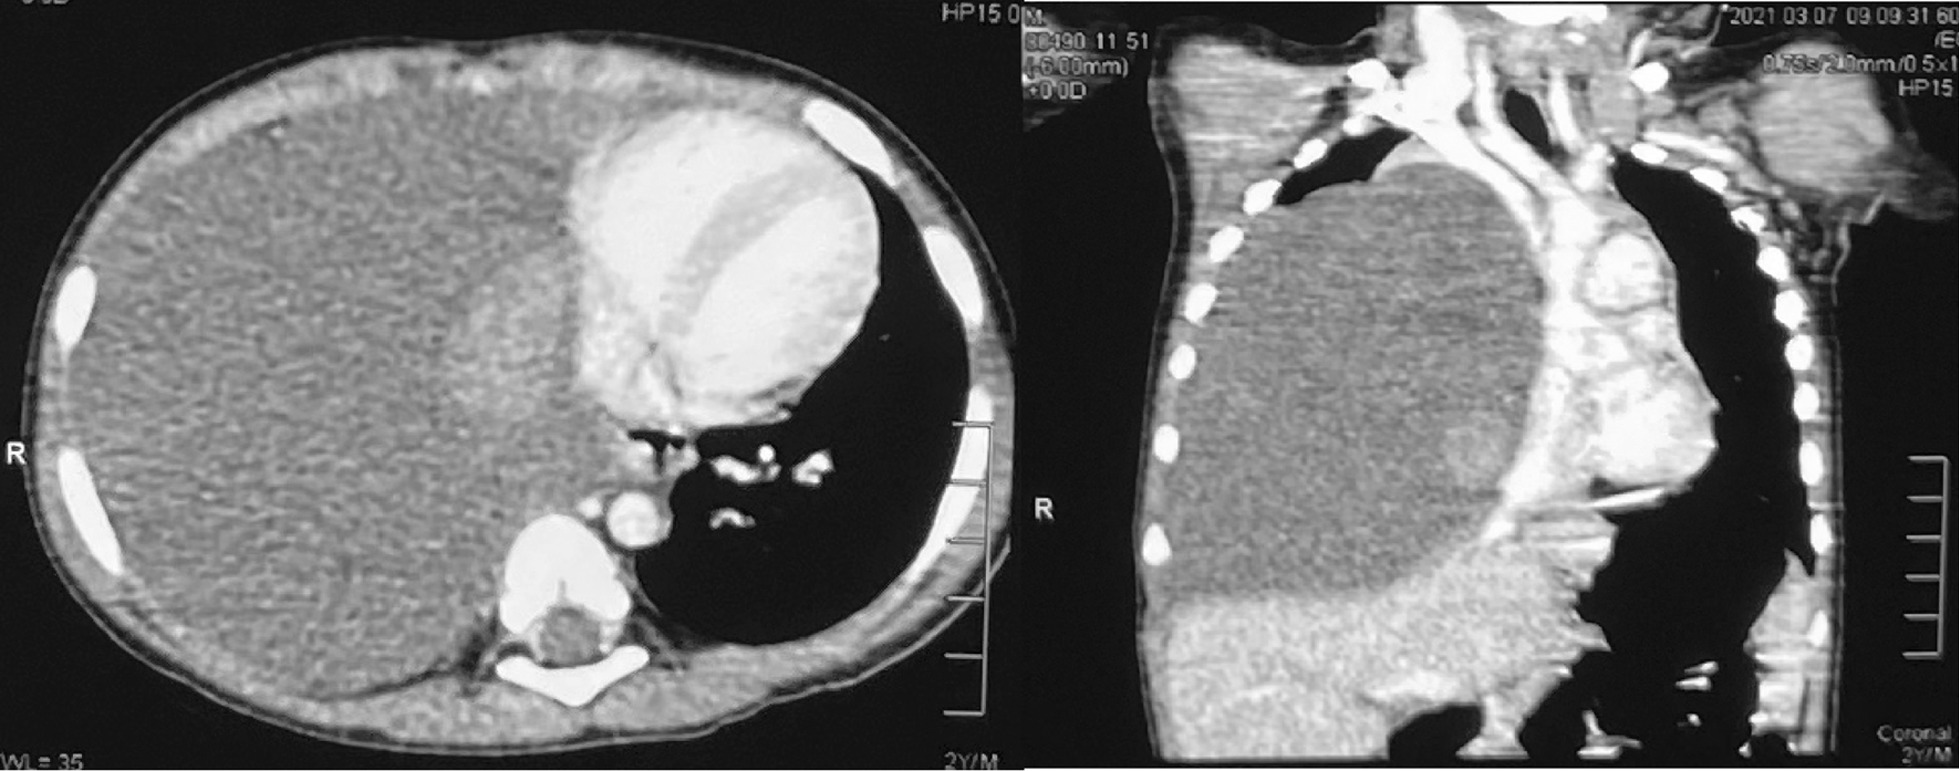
Contrast-enhanced CT scan of the chest showing a large multiloculated extrapulmonary cystic lesion (measuring 11.5 cm × 11.3 cm × 9.5 cm) involving the right hemithorax with a mediastinal shift towards the left and mass effect and displacement of the right-side mediastinal structures. The right lung is compressed superiorly, and the right main pulmonary artery is narrowed
